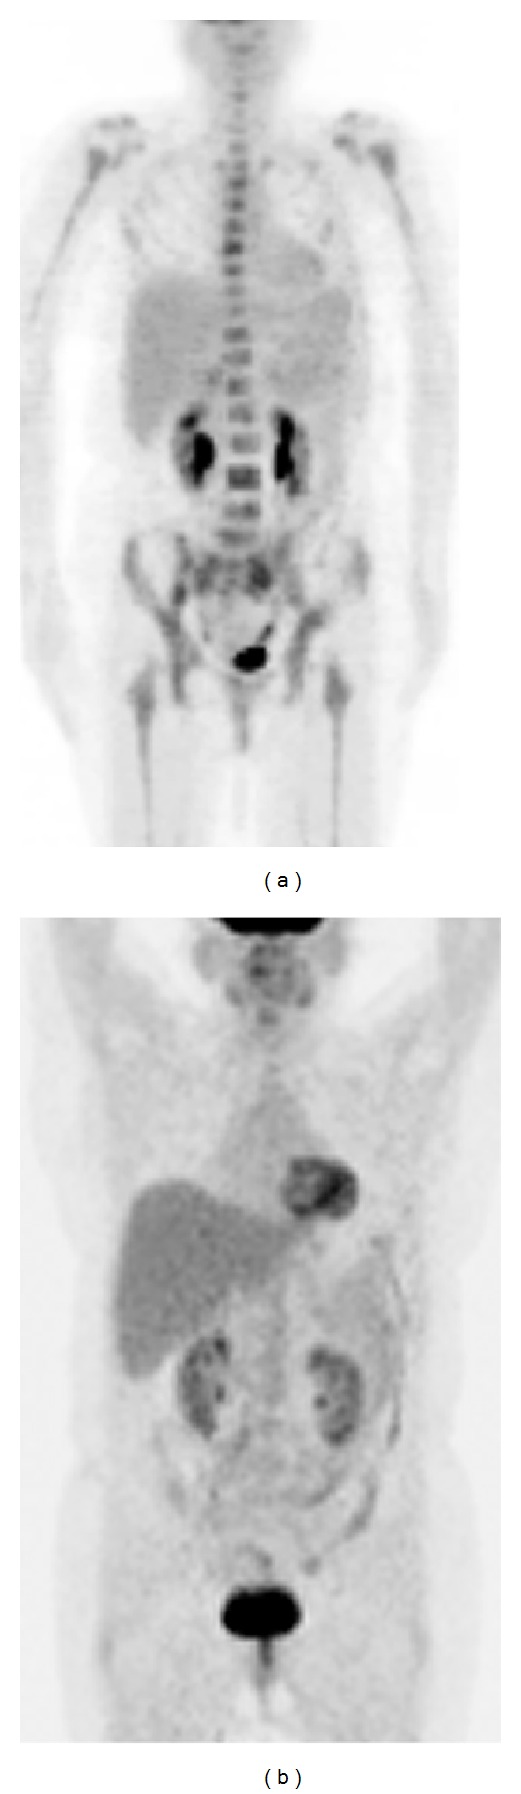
(a) An ^18^F-fluorodeoxyglucose positron emission tomography (FDG-PET) scan showing disseminated bone marrow uptake at diagnosis. (b) FDG-PET scan after autologous stem cell transplantation showing the resolution of FDG uptake.
